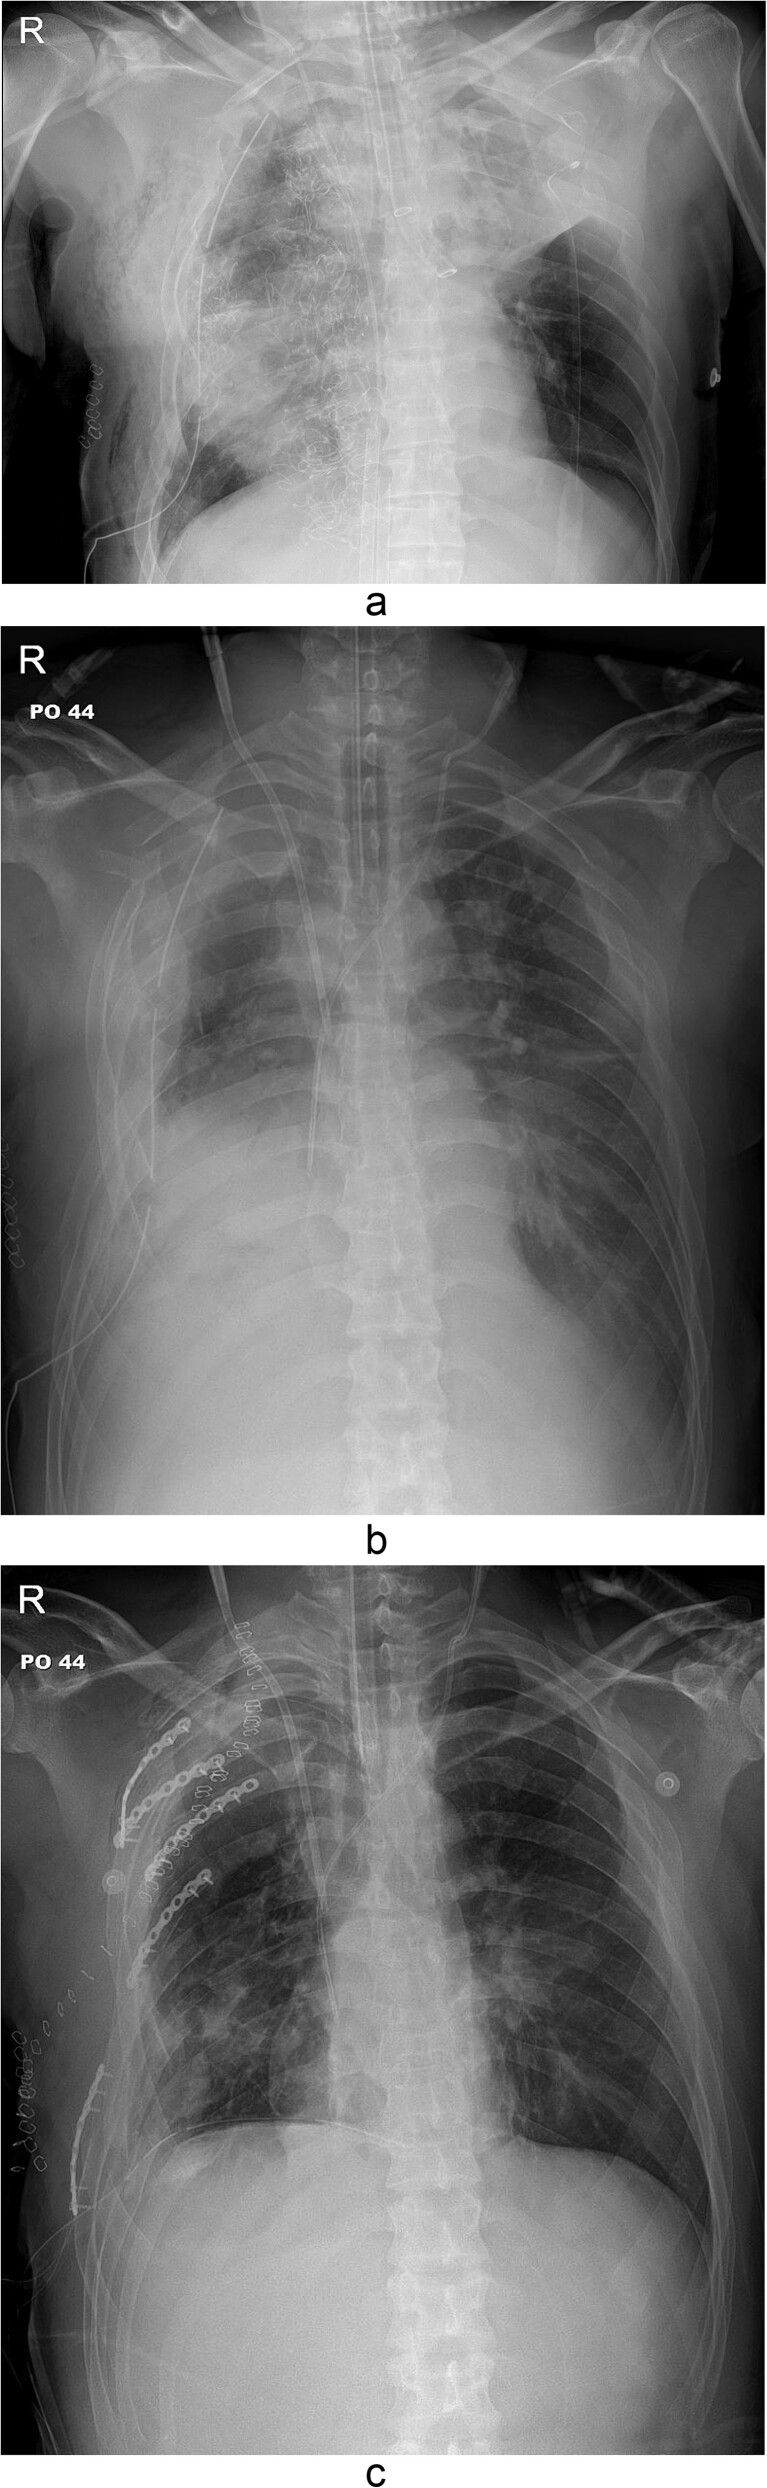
Simple radiographs obtained after each operation. (a) Radiograph after the first operation. Gauze, which is visible as a wavy white line, was packed in the right thoracic cavity. Multifocal opacity was seen in the right lung, and there was left upper lung atelectasis. ECMO catheters were placed in the IVC. (b) Radiograph following the second operation. The right ribcage is narrowed due to displaced fractured ribs. (c) Radiograph after the third operation. Five plates were applied to the fractured ribs.
